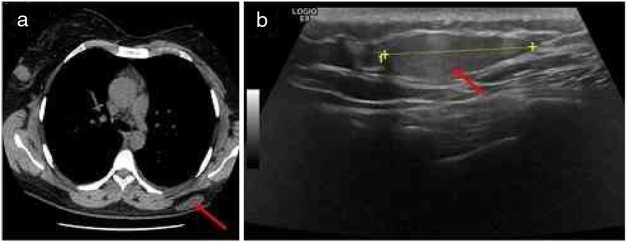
Imagiological features of the tumor: A, High-resolution thoracic computed tomography revealing a large, low-density, subcutaneous nodule in the left dorsal region (red arrow); B, Ultrasound revealing a well-defined, slightly hyperechoic, subcutaneous mass in the left dorsal region (red arrow).
